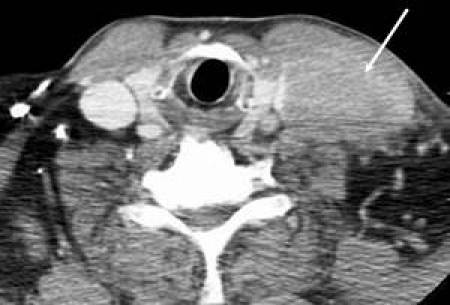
Axial CT scan of the neck showing a left supraclavicular mass that takes contact with the left jugular vein (narrowed and infiltrated) and the left sternocleidomastoid muscle.
